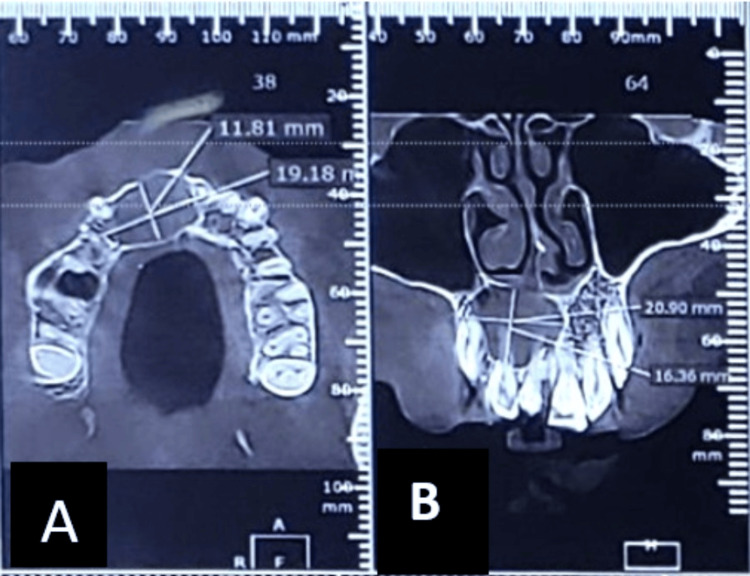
Preoperative CBCT (A) Axial and (B) coronal planes showing expansion of labial and palatal cortical plates. CBCT: Cone beam computed tomography.
